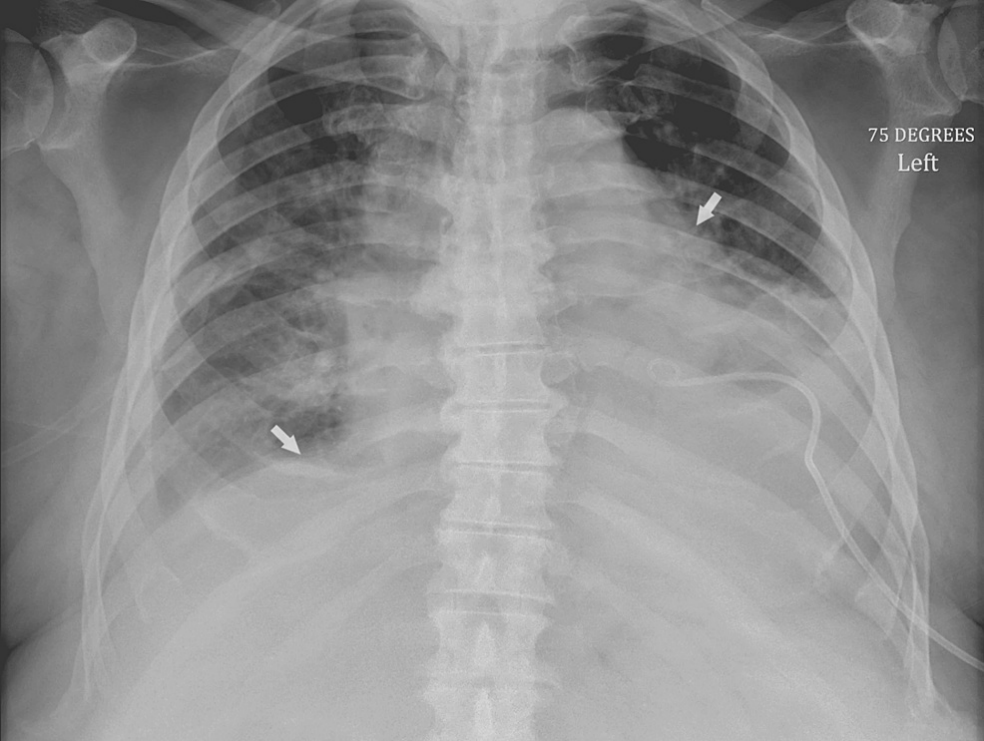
Chest X-ray showing the bilateral pleural effusion White arrows: bilateral pleural effusion
